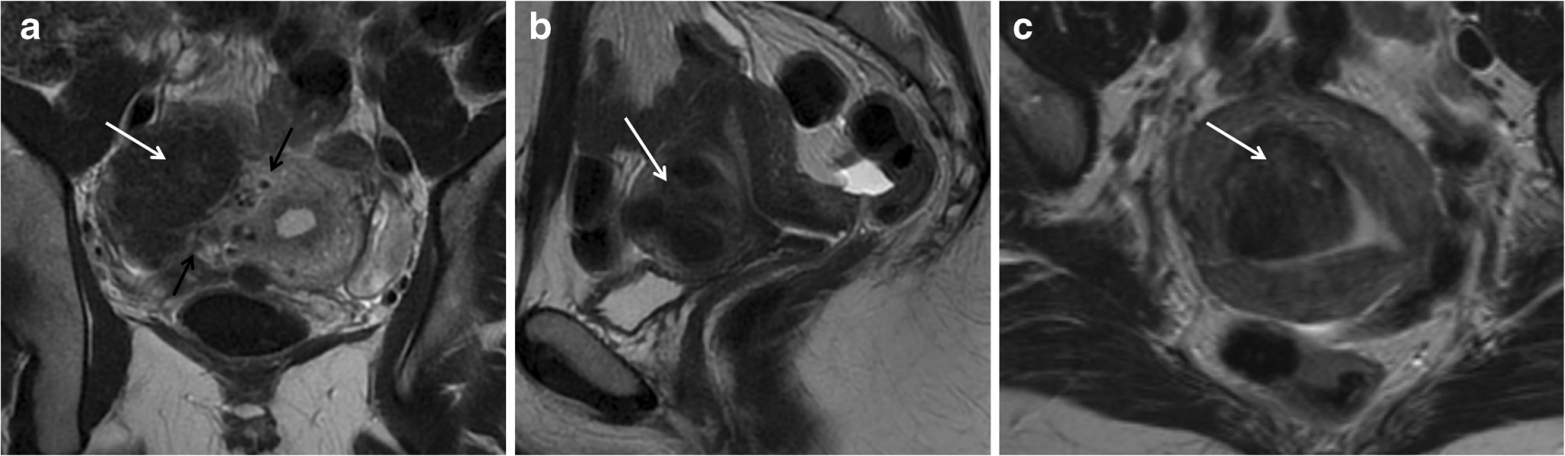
Leiomyoma FIGO classification according to LM location: (a) Axial oblique T2-weighted image showing a subserosal leiomyoma being less than 50% intramural (MRI FIGO 6) (white arrow). Note the bridging vessels feeding the lesion (black arrow). (b) Sagittal T2-weighted image showing an intramural leiomyoma (MRI FIGO 4). (c) Axial oblique T2-weighted image shows a submucosal leiomyoma being less than 50% intramural (MRI FIGO 1)
